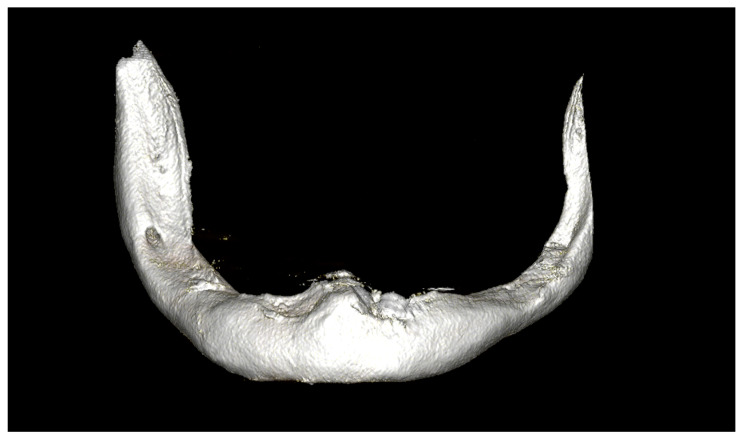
CBCT scan of severely atrophic lower jaw.
